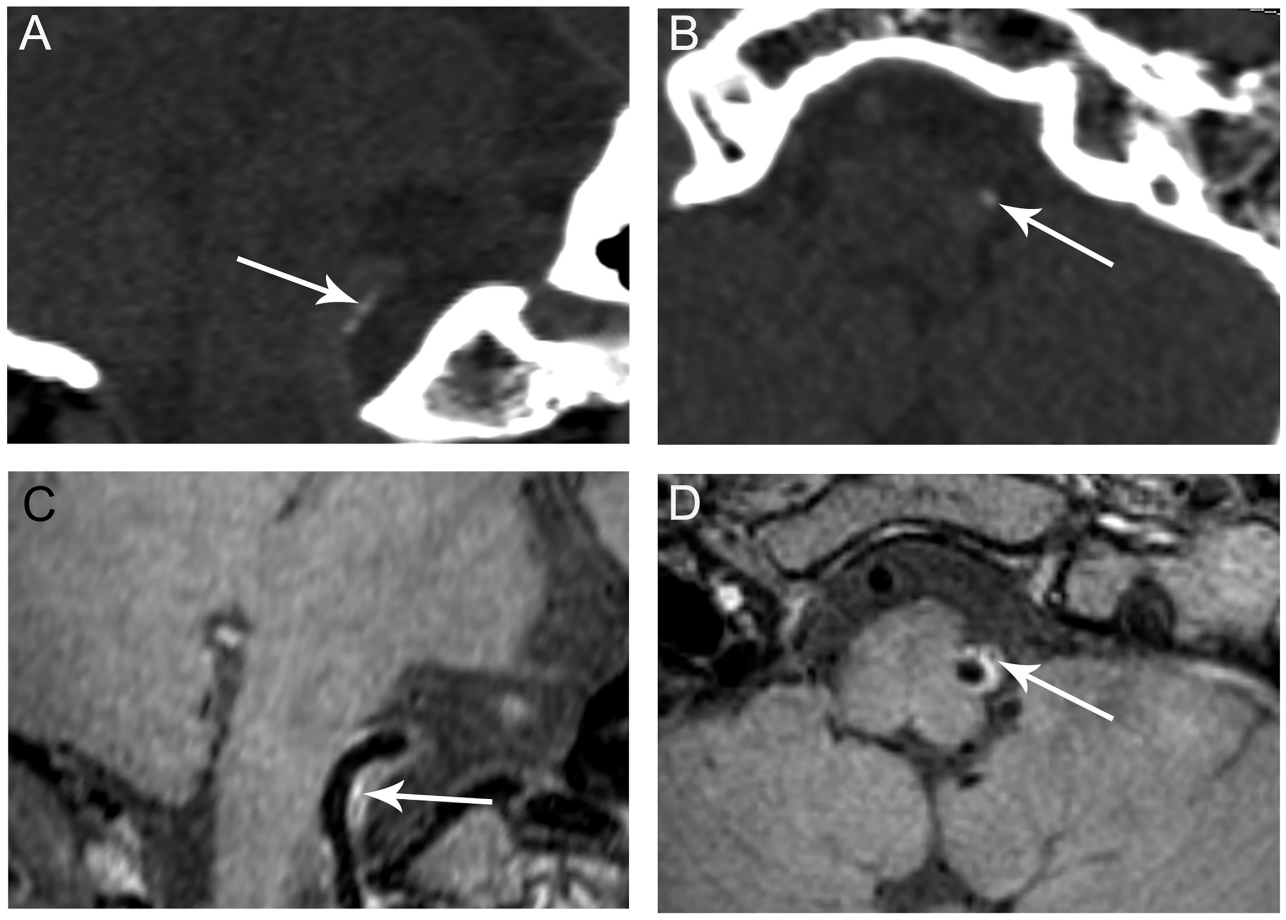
Representative images of predominant intimal VA calcification. CT shows a small clustered calcification on the long (A, arrow) and short axes (B, arrow) of the right VA indictive of intimal calcification. The calcification corresponds to a hypointensity area (C,D, arrows) within an atherosclerotic plaque observed on VWMRI.
